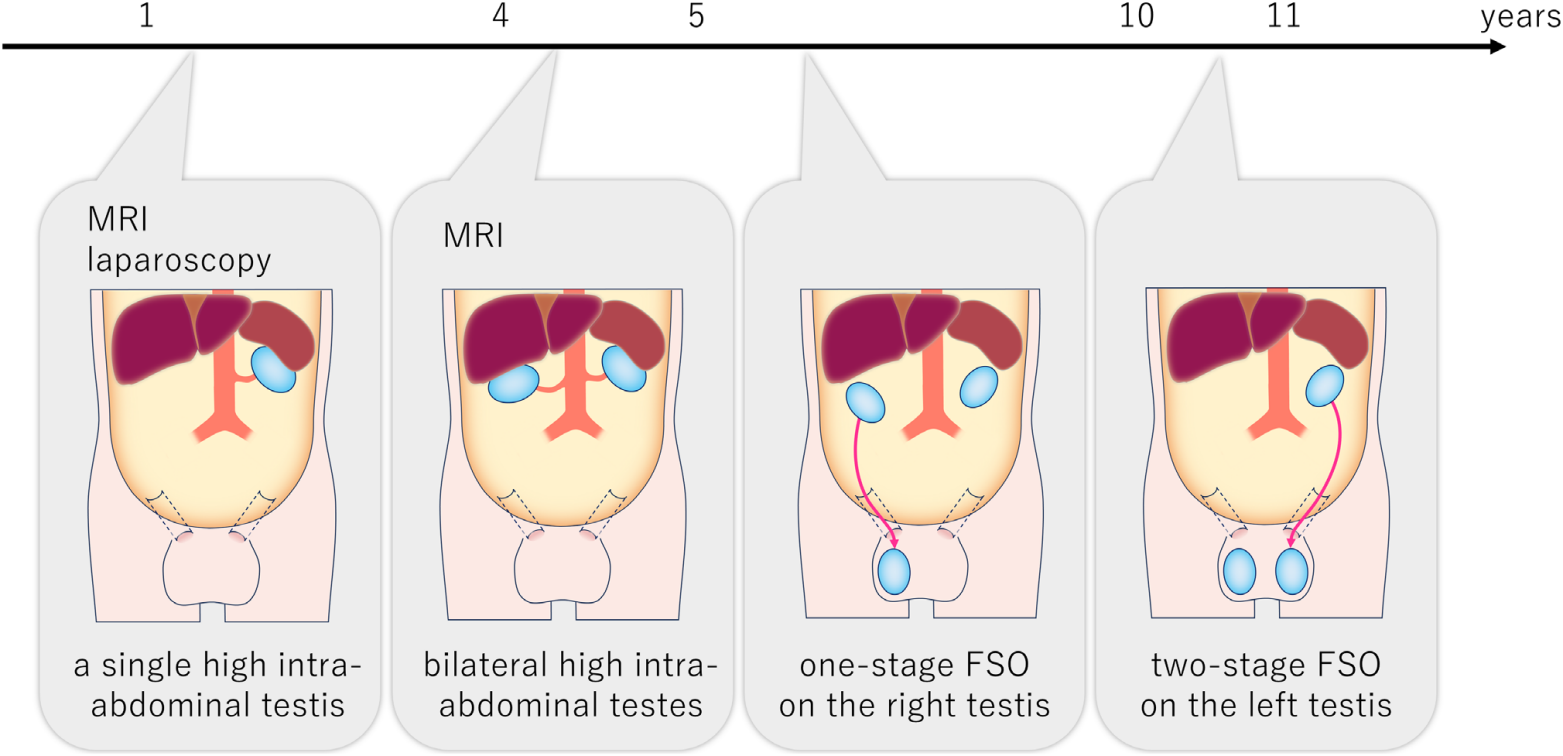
Flow of treatment for this case. First, the patient was diagnosed as monorchism by MRI and laparoscopy, so he was followed up without orchiopexy. But following MRI showed a left testis, so it was diagnosed as bilateral high intra‐abdominal testes. Multistage FSO was performed.
